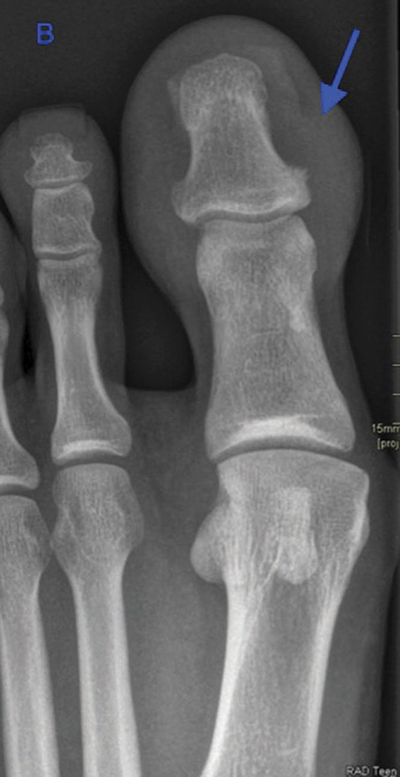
Conventional radiograph of the left foot shows non-specific soft tissue swelling and subtle irregular delineated bone proliferation medially at the distal phalanx (arrow).
